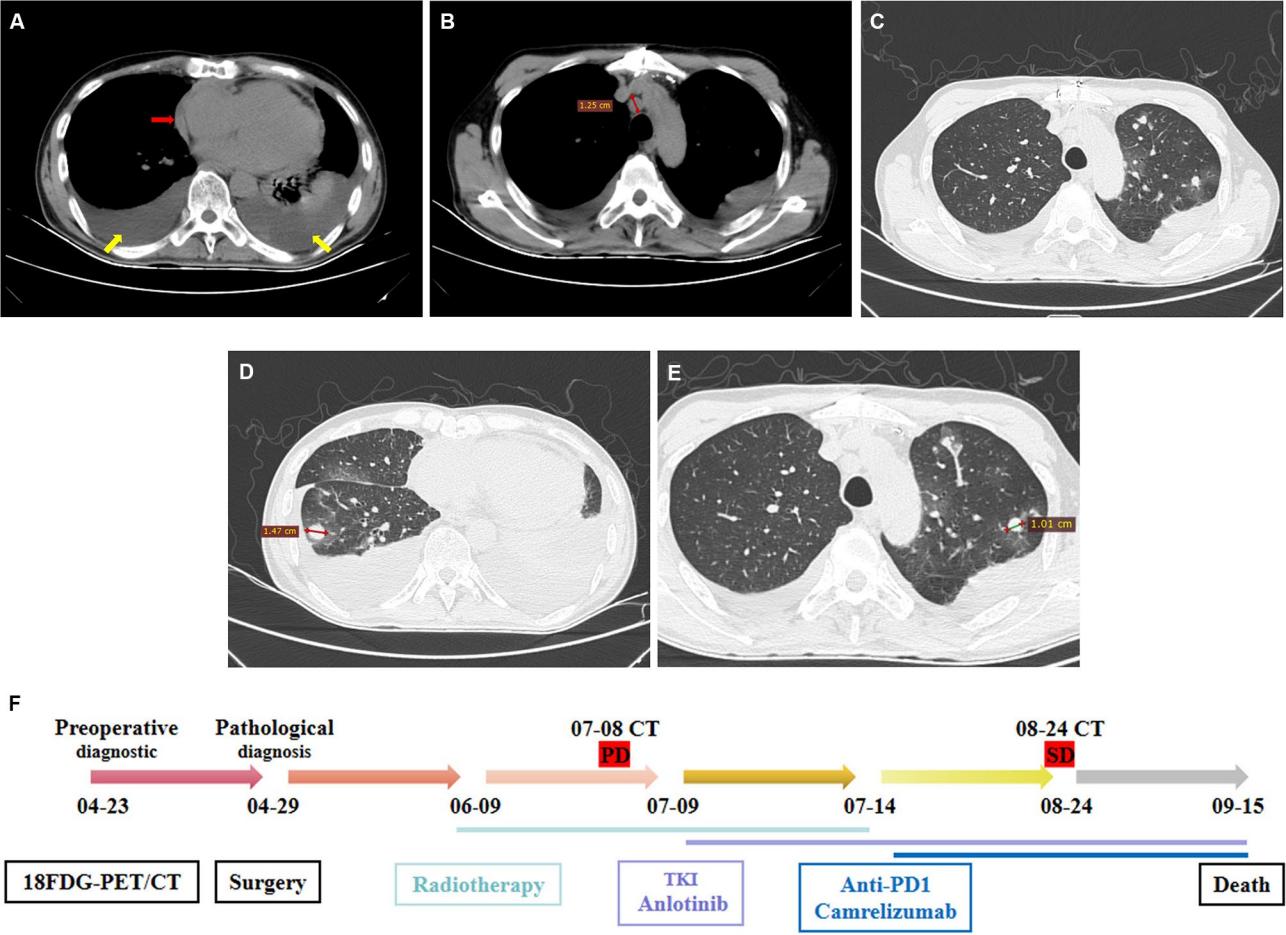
Chest computed tomography (CT) scan to evaluate the efficacy. (A–D) Images of disease progression during postoperative radiotherapy. (A) Bilateral pleural and pericardial effusion (shown in yellow and red arrows, respectively). (B) Station 4R was enlarged with a long diameter of about 1.25 cm. (C) Multiple nodules in both lungs (highly suspected metastasis). (D) The longest diameter of pulmonary nodules was about 1.47 cm. (E) After immunotherapy, bilateral pulmonary nodules could be observed. The longest diameter was approximately 1.01 cm. (F) Timeline
